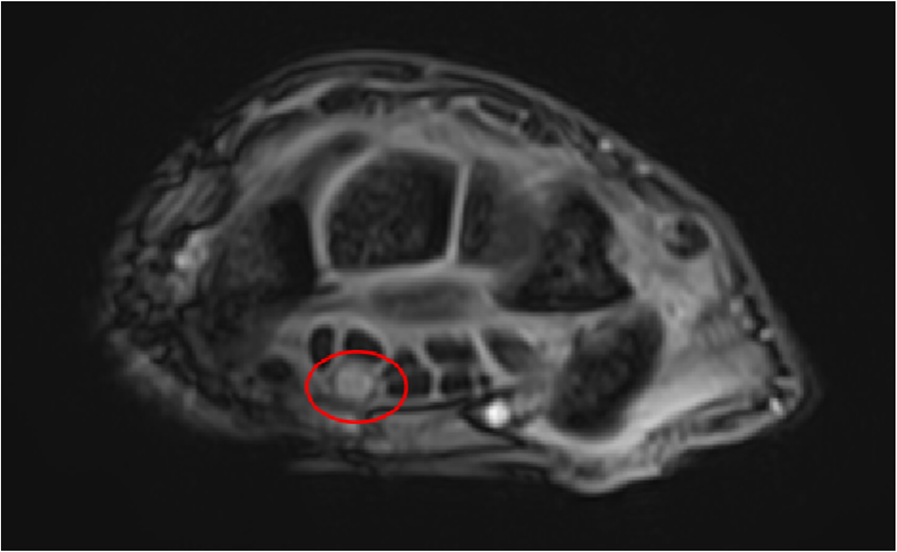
Magnetic resonance imaging of the left wrist. The red circle indicates the left median nerve. Magnetic resonance imaging shows swelling of the left median nerve and no hematoma
